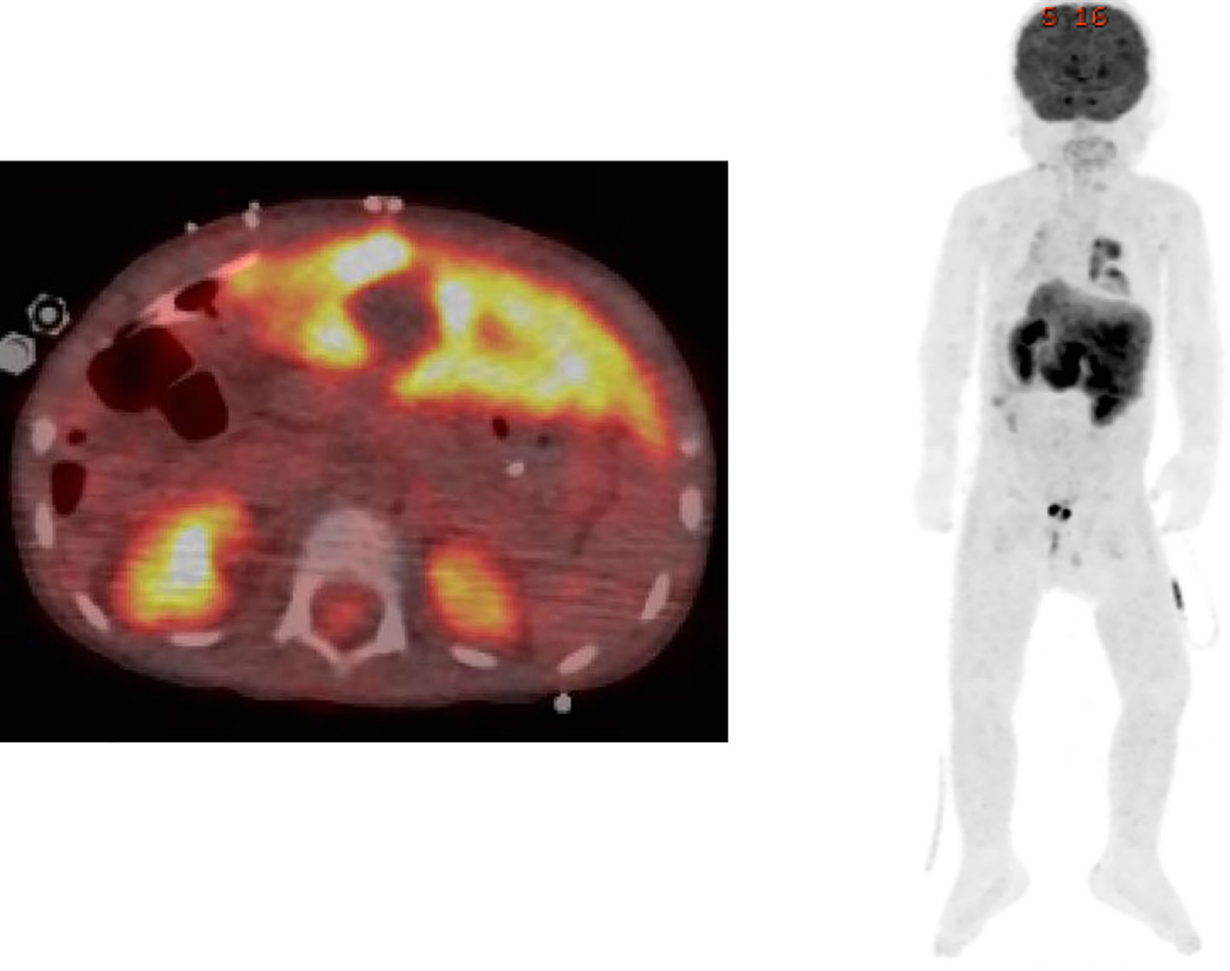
FDG-PET/CT image showing that abnormal uptake is confined to the liver.
